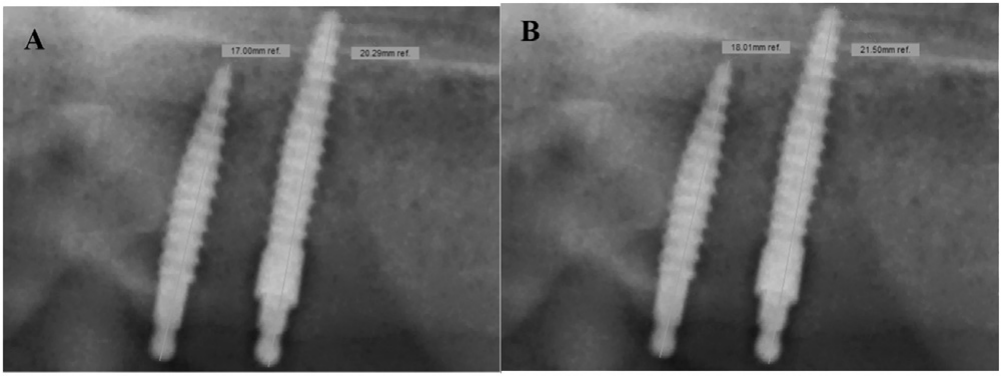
Calibration to the real implant length for a uncollared mini-implant (left side 3A), screw length 13 mm, after correction at the left radiograph) and a collared mini-implant (right side 3B), screw length 15 mm, after correction at the right radiograph).
